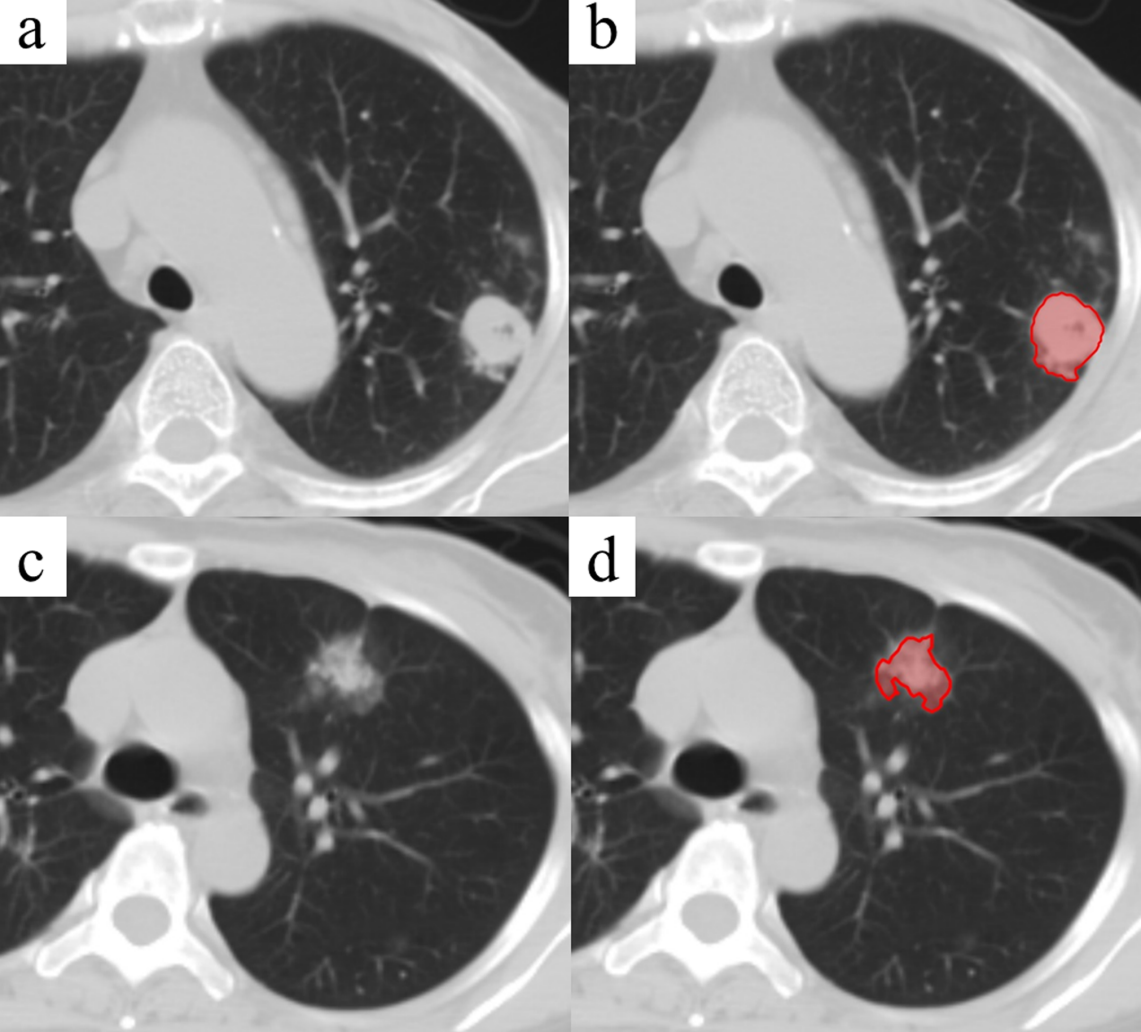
Examples of lung lesion segmentation. Original CT image (a) and target segmentation (b) of a benign lung lesion (tuberculosis) in patient’s left upper lobe. Another original CT image (c) and target segmentation (d) of a malignant lung tumor (adenocarcinoma) in patient’s left upper lobe.
